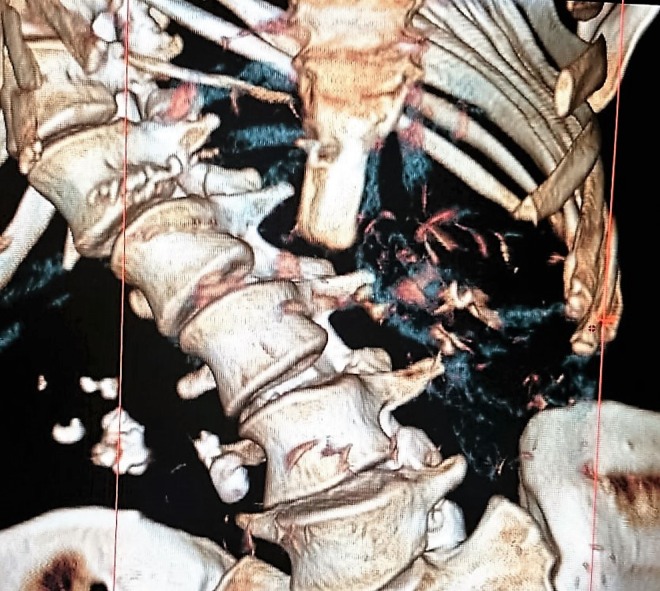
Abdominal CT that shows right stones and severe kyphoscoliosis (reconstruction, front vision). CT, computed tomography.
